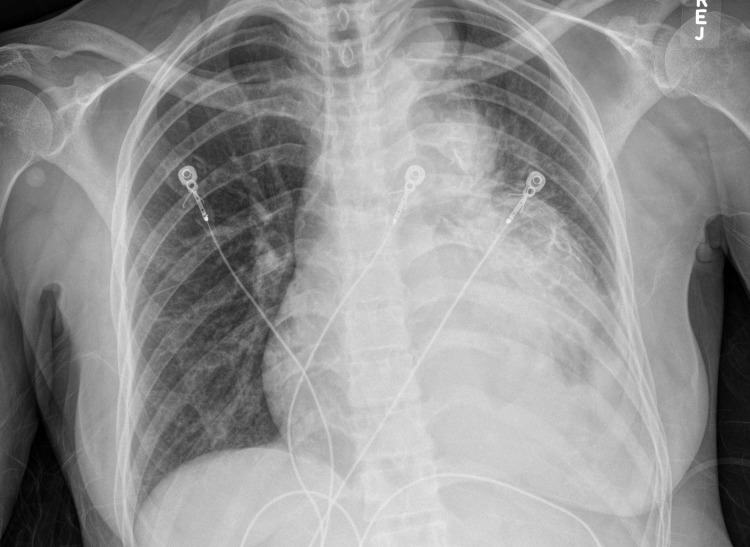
Chest X-ray showing cardiomegaly and left sided pleural effusion
